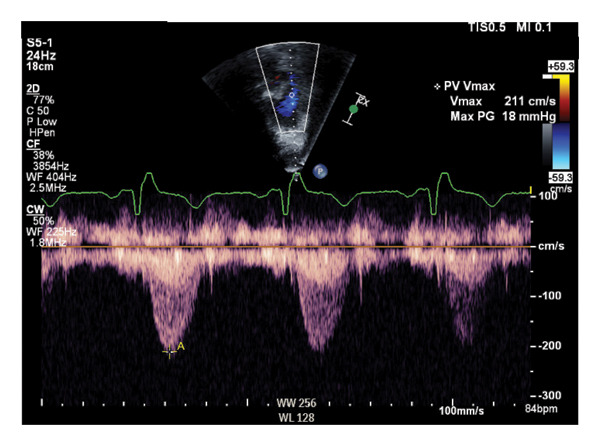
Continuous wave Doppler on TTE demonstrating a peak systolic gradient of 18 mm Hg across RVOT.
